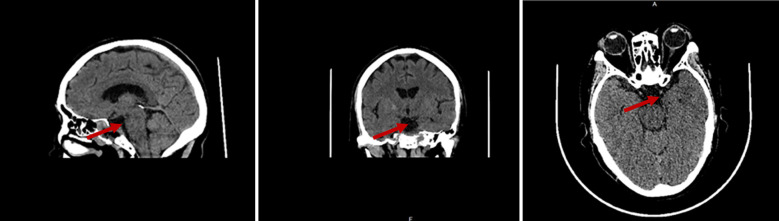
First postoperative follow-up. On postoperative day 5, plain cranial CT scan with three-dimensional reconstruction shows: Postoperative changes in the suprasellar-sellar region; the previously observed mass shadow is no longer visible, with localized irregularity of the adjacent bone.
